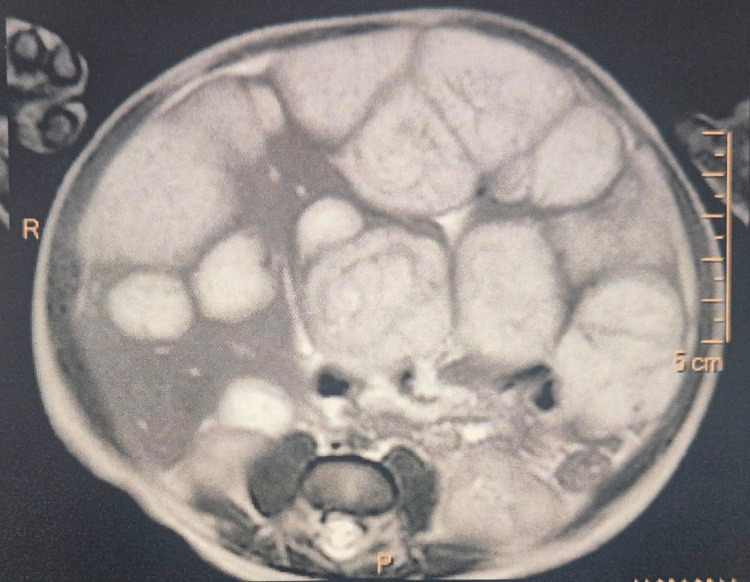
Abdominopelvic MRI showing evidence of infantile hepatic hemangiomatosis.
